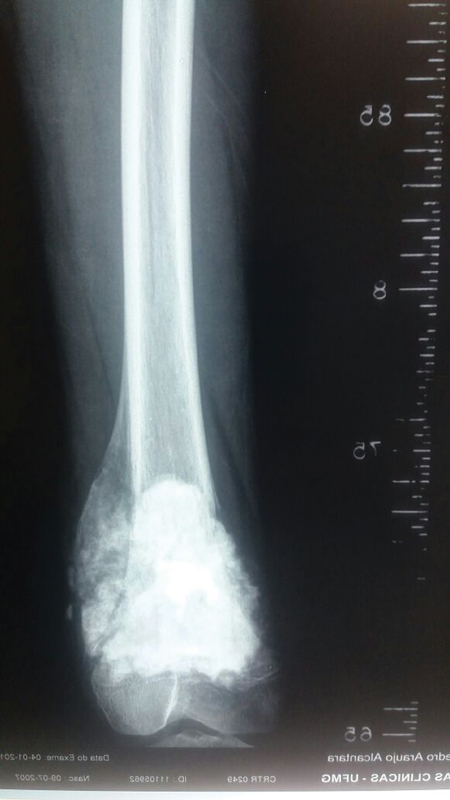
Radiographic appearance of conventional osteosarcoma. Source: Author's personal file.
